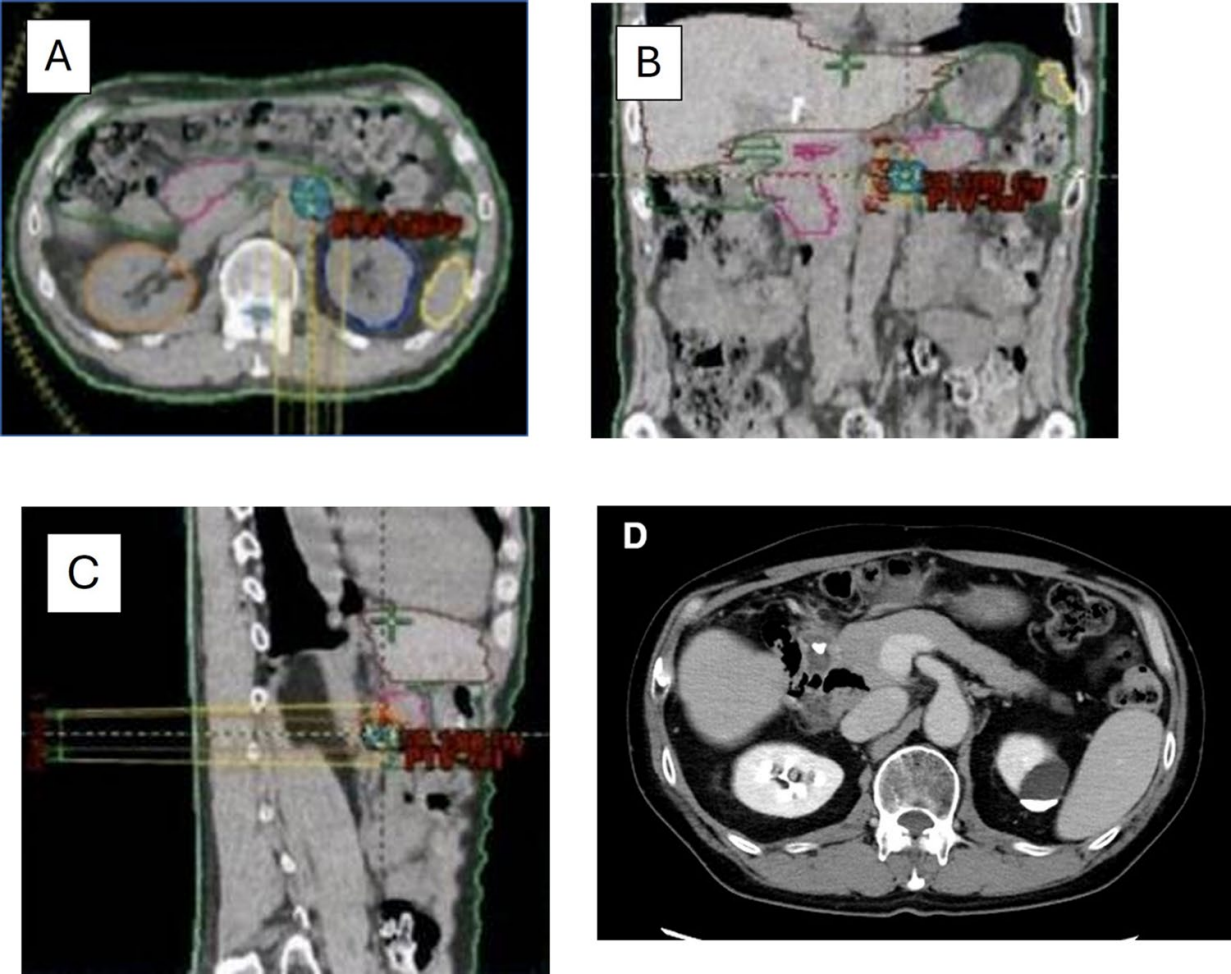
Radiotherapy planning CT. A Axial view, B coronal view, C sagittal view and D CT (axial view) at 50 months after surgery. There was no lymph node swelling
